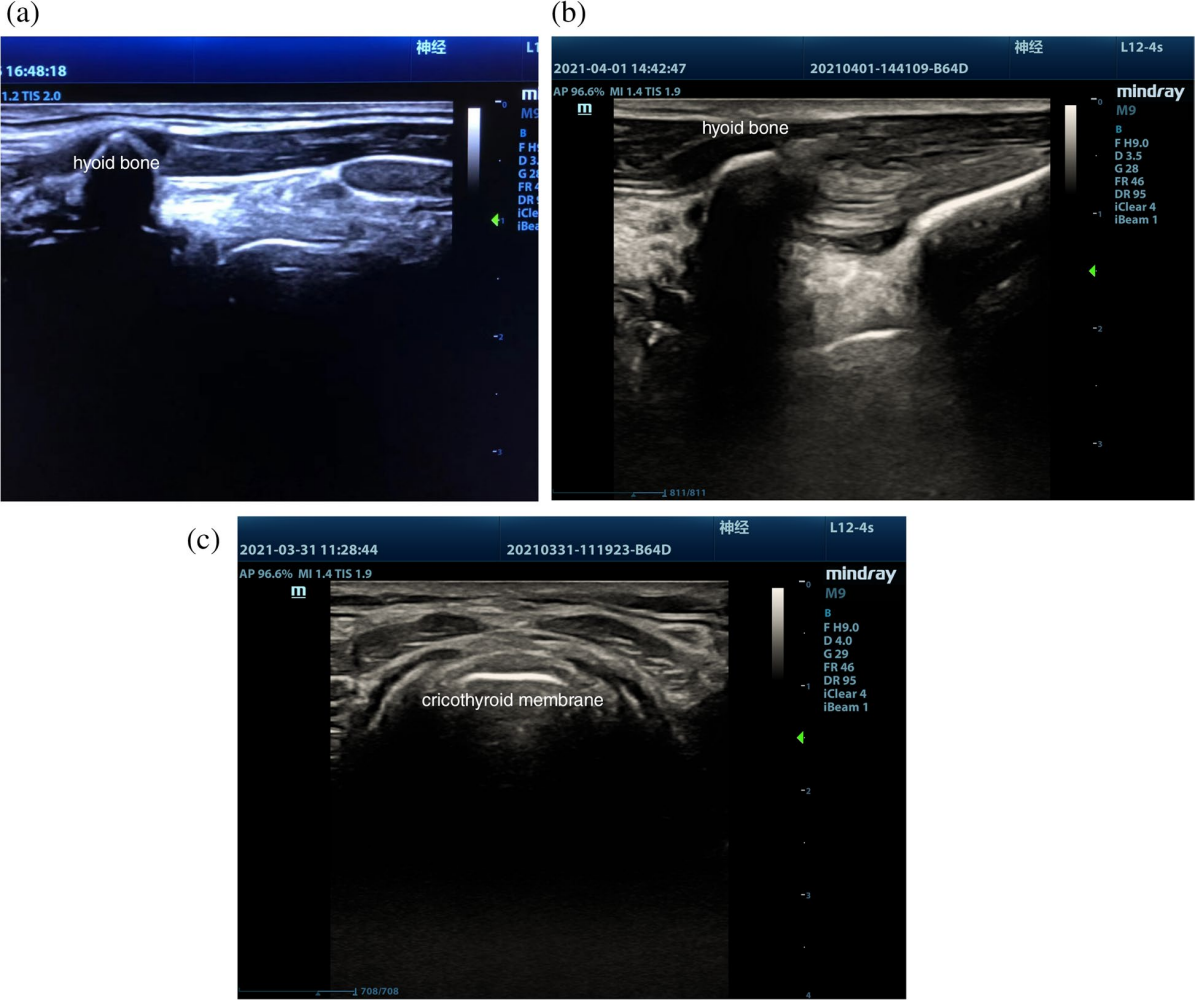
Ultrasound-guided SLN block and transtracheal injection. Note: a The tissue structure around the SLN under sagittal ultrasound, b the sagittal out-of-plane puncture under ultrasound. 2 ml of 2% lidocaine solution was injected into the thyroglossal membrane. c The location of the cricothyroid membrane under coronal ultrasound
